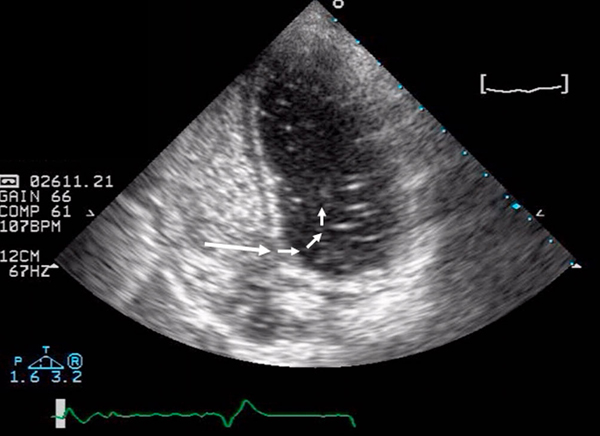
Contrast ('bubble') transthoracic echocardiogram showing the presence of a patent foramen ovale (large arrow) with a right-to-left shunt (small arrows).
